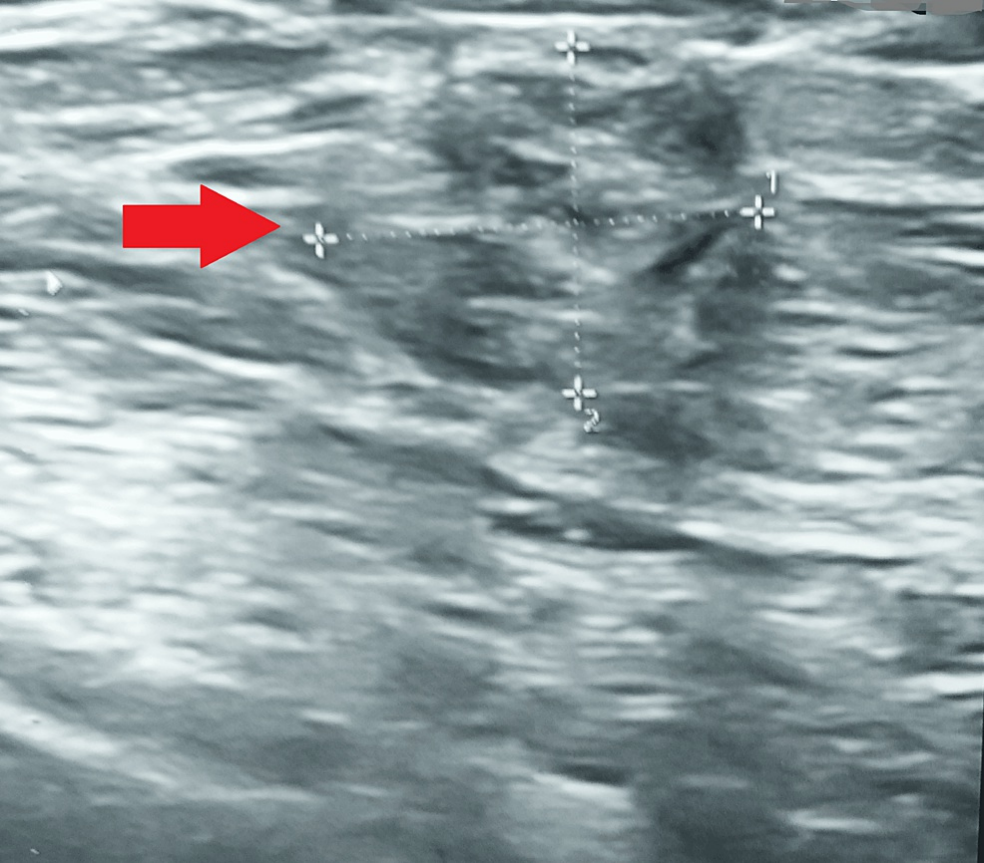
A chest ultrasound suggestive of an irregular collection
